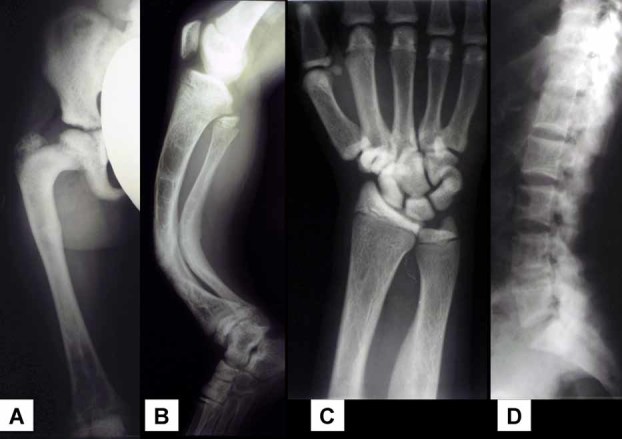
Radiographs of patient 1 obtained between 1952 and 1969. (*A*) Severe coxa vara (12 years) and (*B*) lower leg deformity (14 years). (*C*) Delayed closure of epiphyses and calcification of the forearm interosseus membrane (18 years). (*D*) Paraspinal calcifications without deformities of the vertebral column (29 years).
